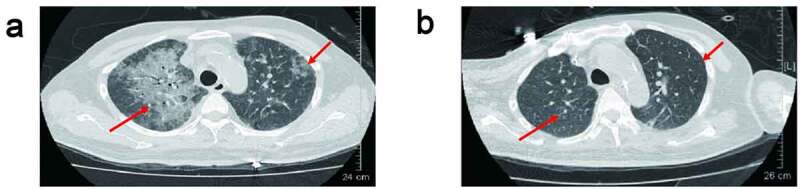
(a) Chest CT of the patient revealed bilateral ground-glass opacity before transferred to our department. (b) Chest CT of the patient revealed bilateral ground-glass opacity absorbed after treatment
